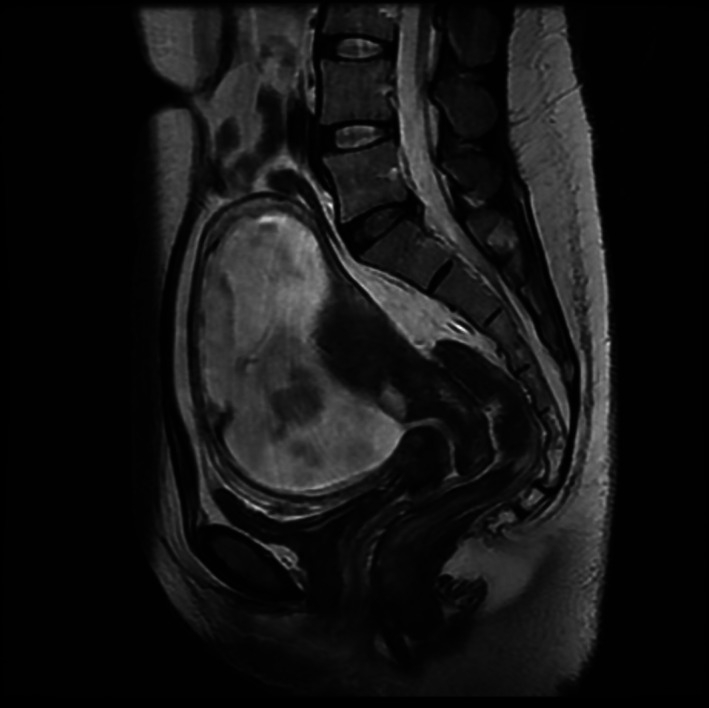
T2‐weighted sagittal magnetic resonance imaging of the pelvic area at 16 weeks' gestation reveals no evidence of remnant cervical cancer
